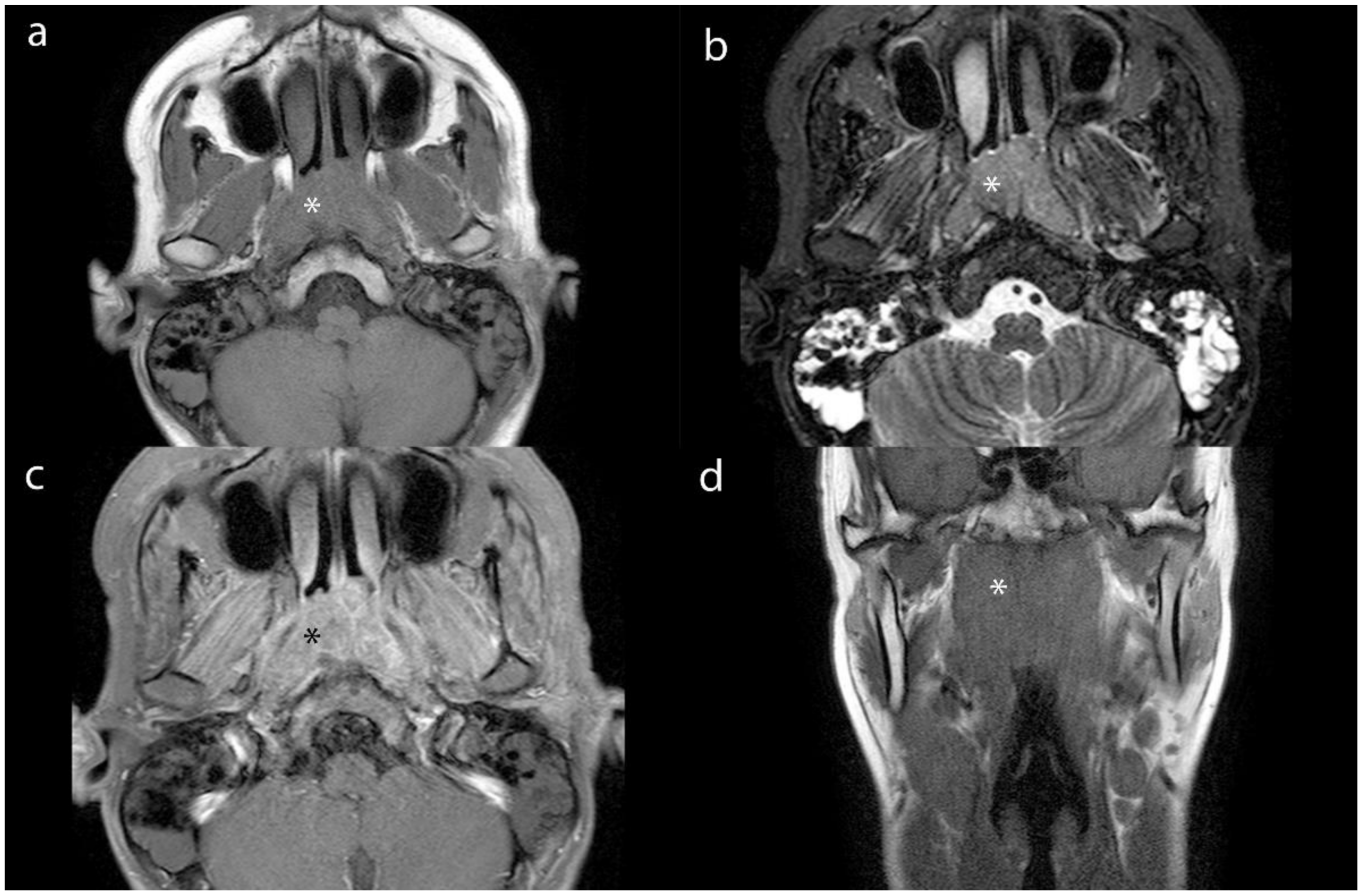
Axial MR images (a-c) and coronal MR image (d) of lesion (*) from a 16-year-old male patient with NPC. (a), (b) and (c) show the T1WI, STIR and contrast- enhanced images. (d) shows the T1WI image.
